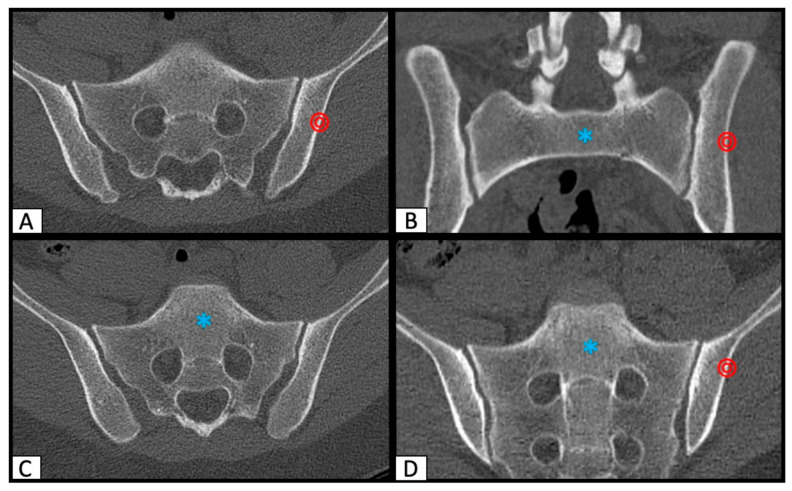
Determination of the perfect safe osseous corridor on axial (A), (C) as well as inlet (B) and outlet (D) orientated CT (computed tomography) scan slices. (A) shows the entrypoint (red circle), (C) the endpoint (blue asterix) for the SI (sacro-iliacal) screw in the axial view. (B) shows the planned position for the SI screw in inlet-, (D) in outlet orientated angulation.
